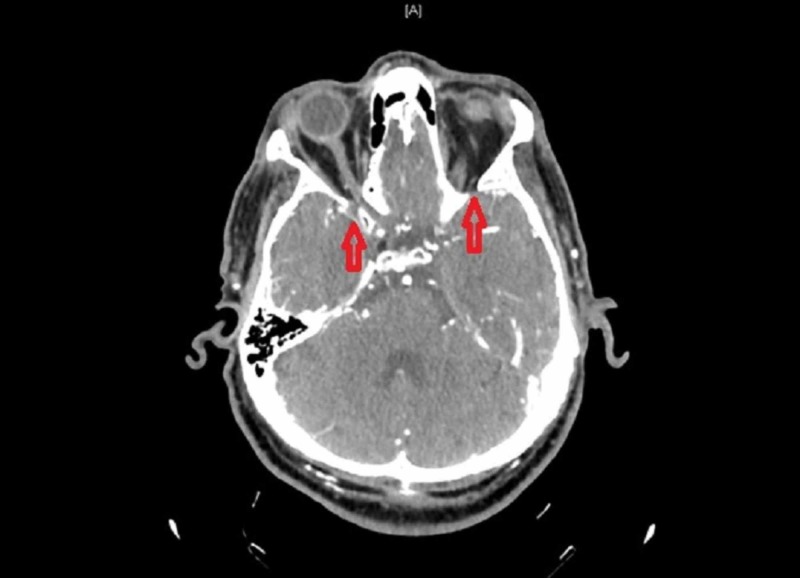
Neck and head CT angiography showed bilateral ICA occlusion ICA, internal carotid artery
